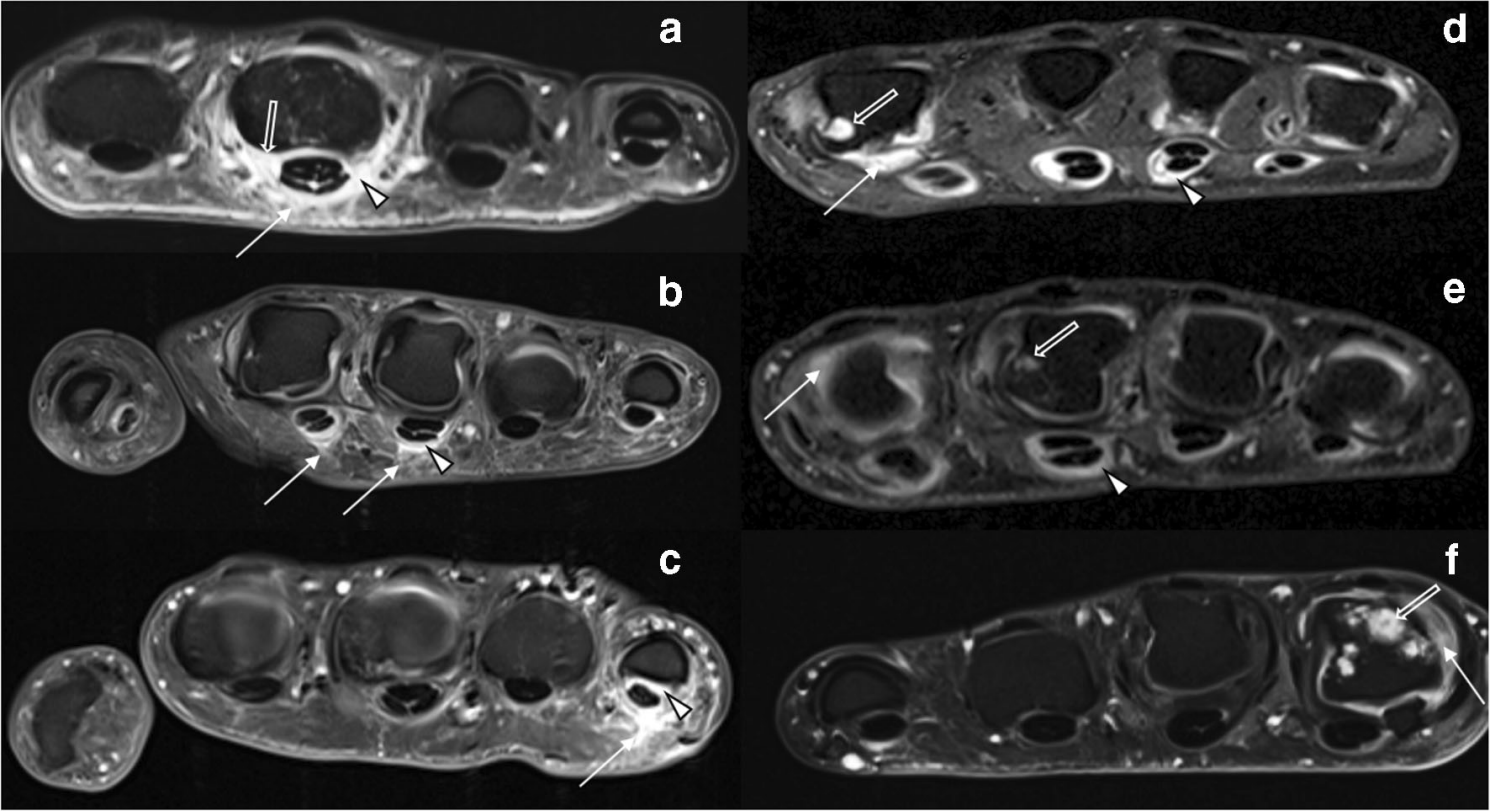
Overview of representative MRI findings in psoriatic arthritis (PsA) and rheumatoid arthritis (RA). Transversal T1w fat-saturated contrast-enhanced sequences of selected MCP joints of three patients each with PsA (a–c) and RA (d–f). a 39-year-old male. Severe periarticular inflammation (white arrow) with additional flexor tenosynovitis (arrowhead) and synovitis (open arrow). b 43-year-old male. Moderate periarticular inflammation (white arrow) with flexor tenosynovitis (open arrow). c 39-year-old female. Severe periarticular inflammation (white arrow) with corresponding flexor tenosynovitis (arrowhead). d 48-year-old female. Widespread synovitis (white arrow), bone erosions (open arrow), and severe flexor tenosynovitis (arrowhead). e 39-year-old male. Bone erosion (open arrow), synovitis (white arrow), and severe flexor tenosynovitis (arrowhead). f 43-year-old male. Multiple bone erosions (open arrow) and synovitis (white arrow). Note the absence of periarticular inflammation in d–f despite significant inflammatory joint changes at the joint level
